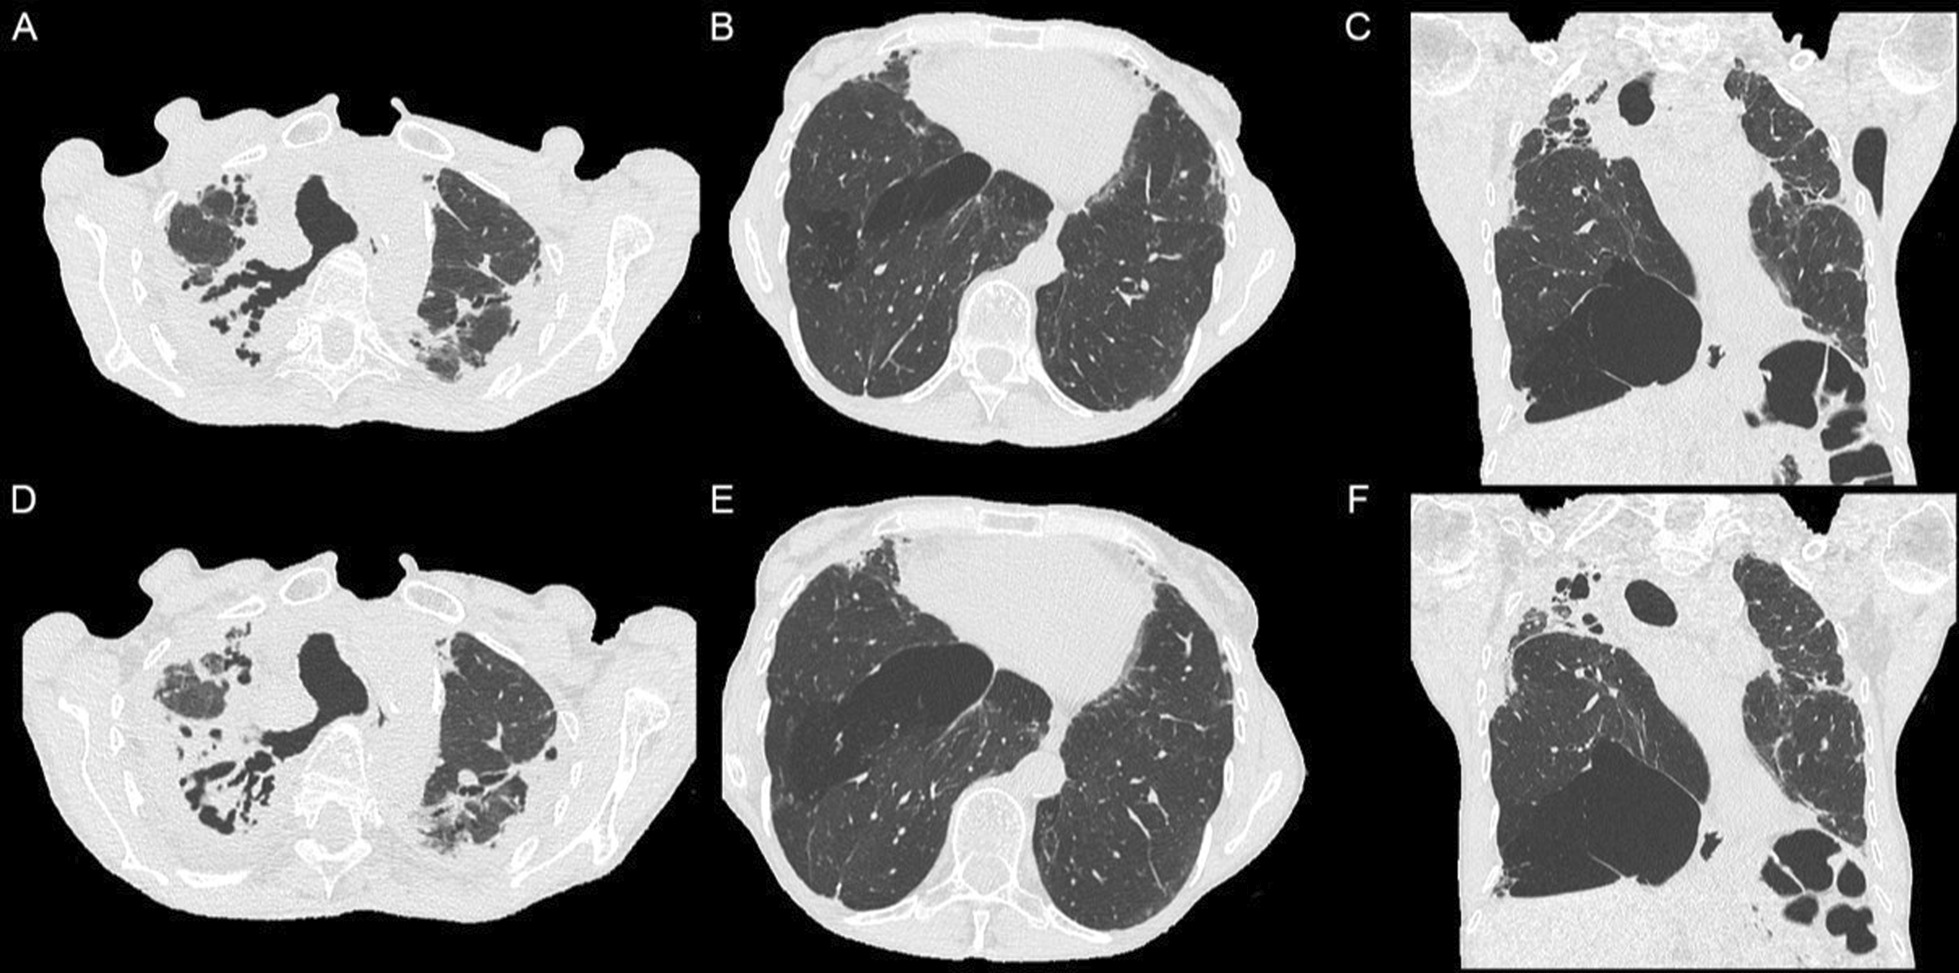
HRCT in a non-smoker patient with PPFE, who received nintedanib as only treatment, demonstrating progression of upper lobe fibrosis (A vs D and C vs F), as well as progression of “compensatory” emphysema in the right base (B vs E and C vs F)
